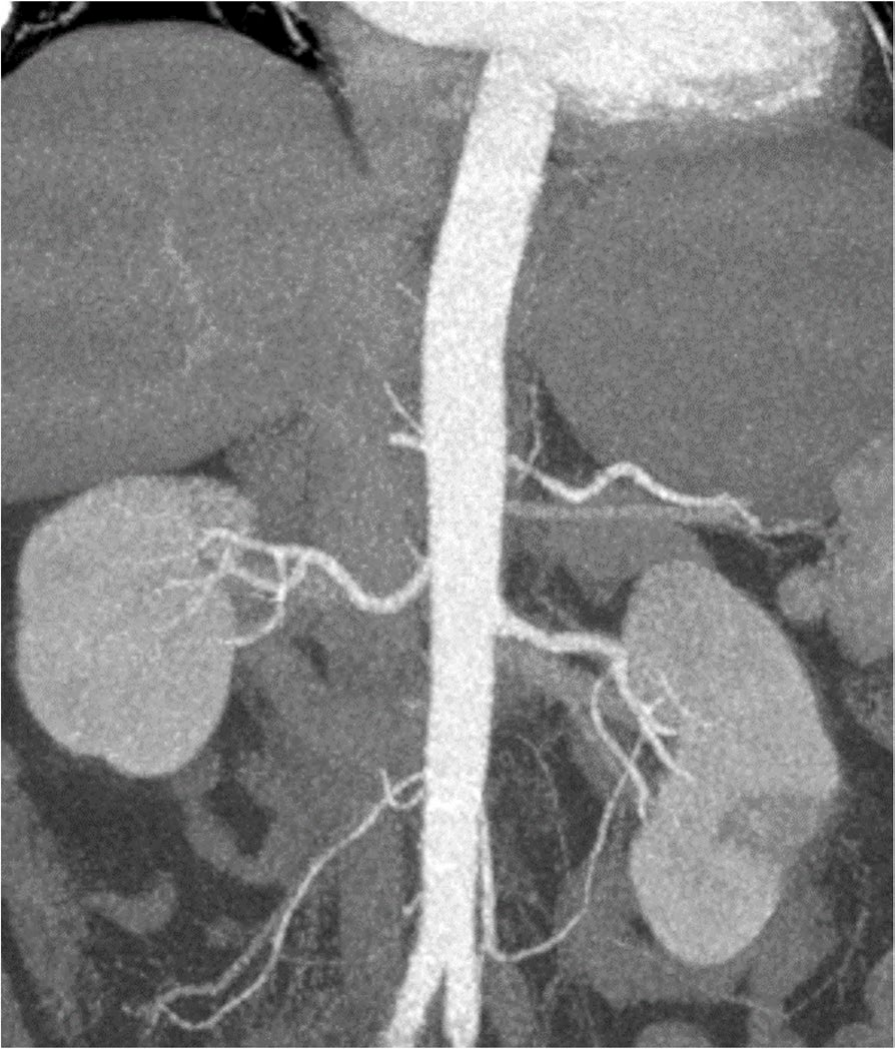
Computed tomography angiography showing a left sided kidney infarct
